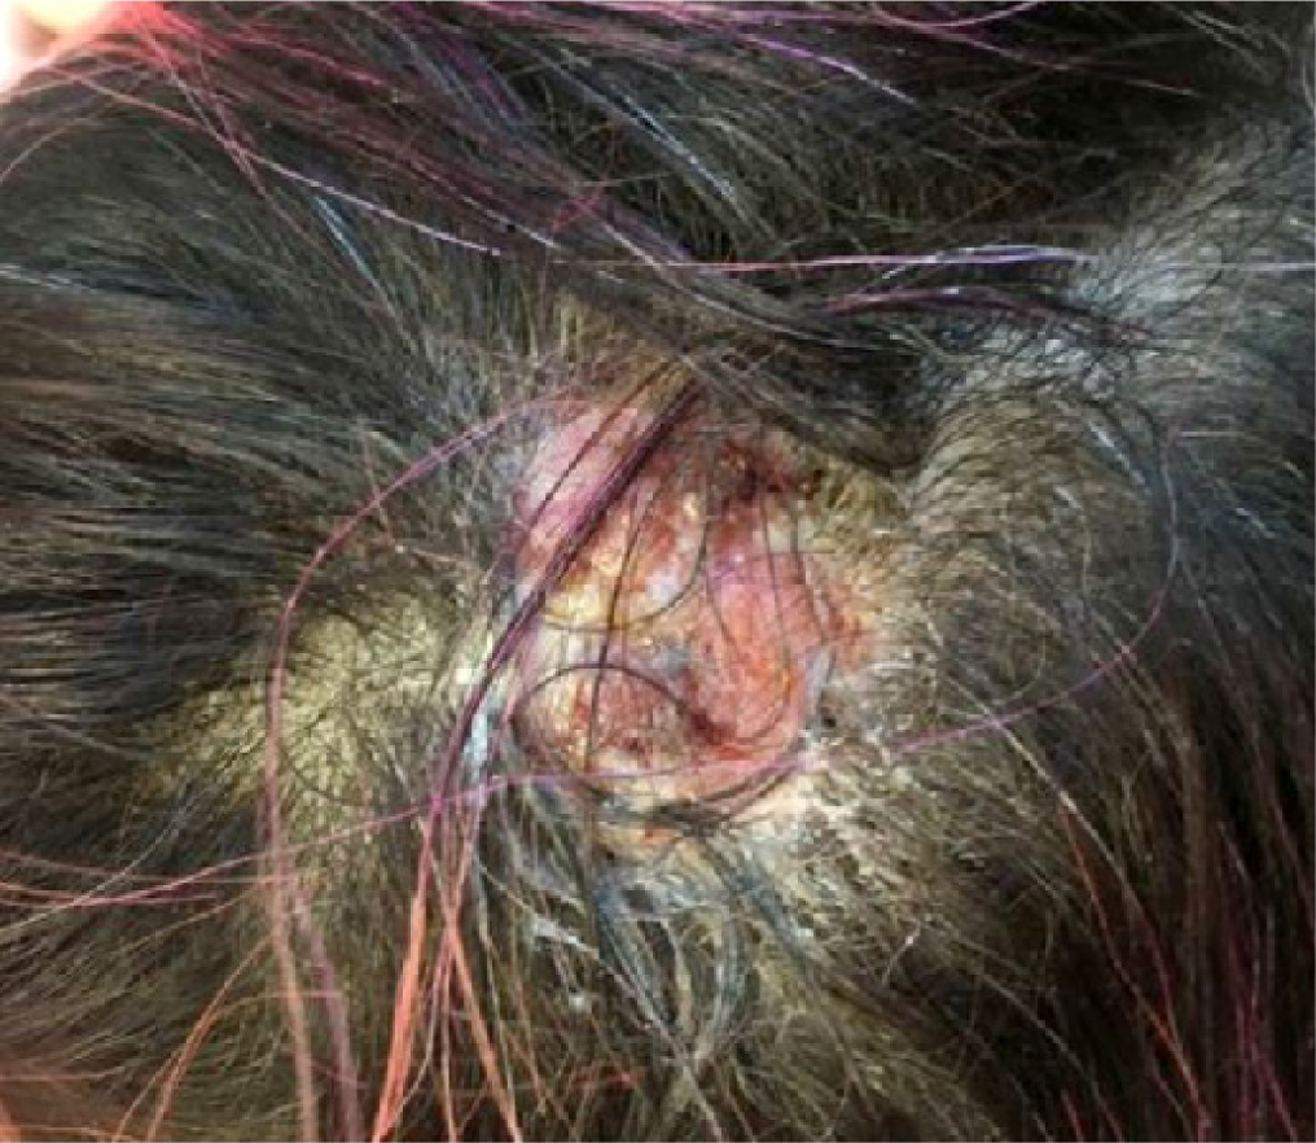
MRI scan of headshowing the location and depth of scalp mets.
